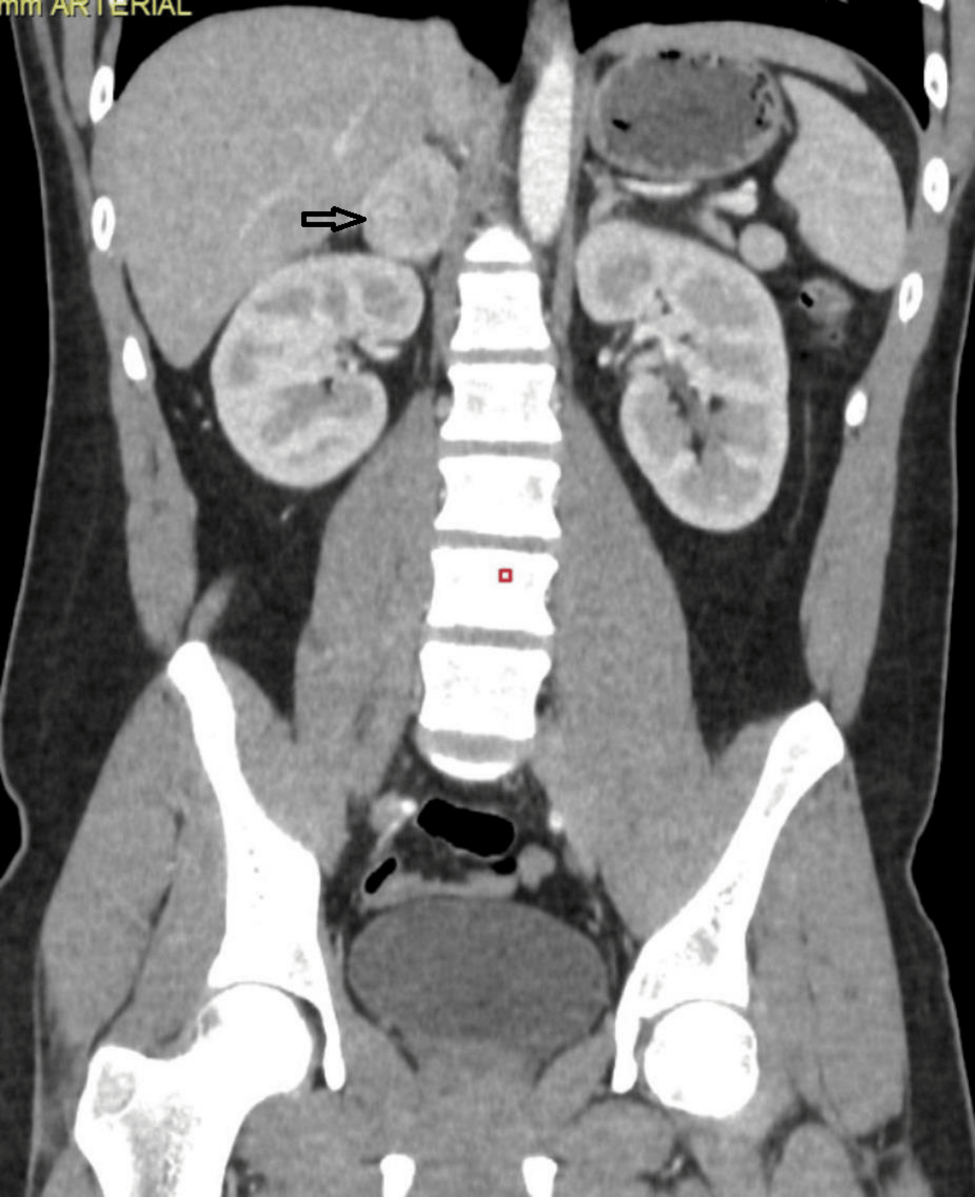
Contrast CT scan of the abdomen and pelvis cross coronal view showing a right adrenal tumor (measured as 4.2cm x 3.4cm x 4.8cm) (black arrow)
